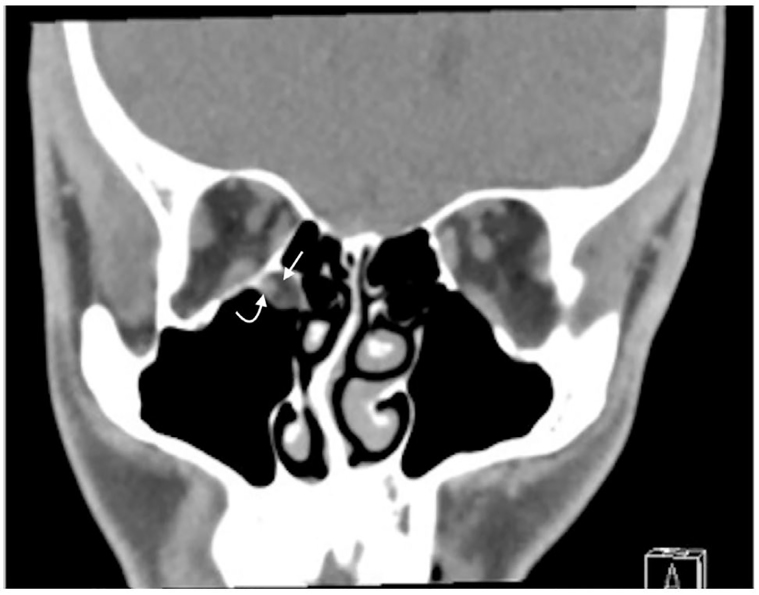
Coronal non-enhanced CT in soft tissue window: Inferior rectus muscle (curved arrow) and orbital fat (straight arrow) in the extra-orbital maxillary antrum.
